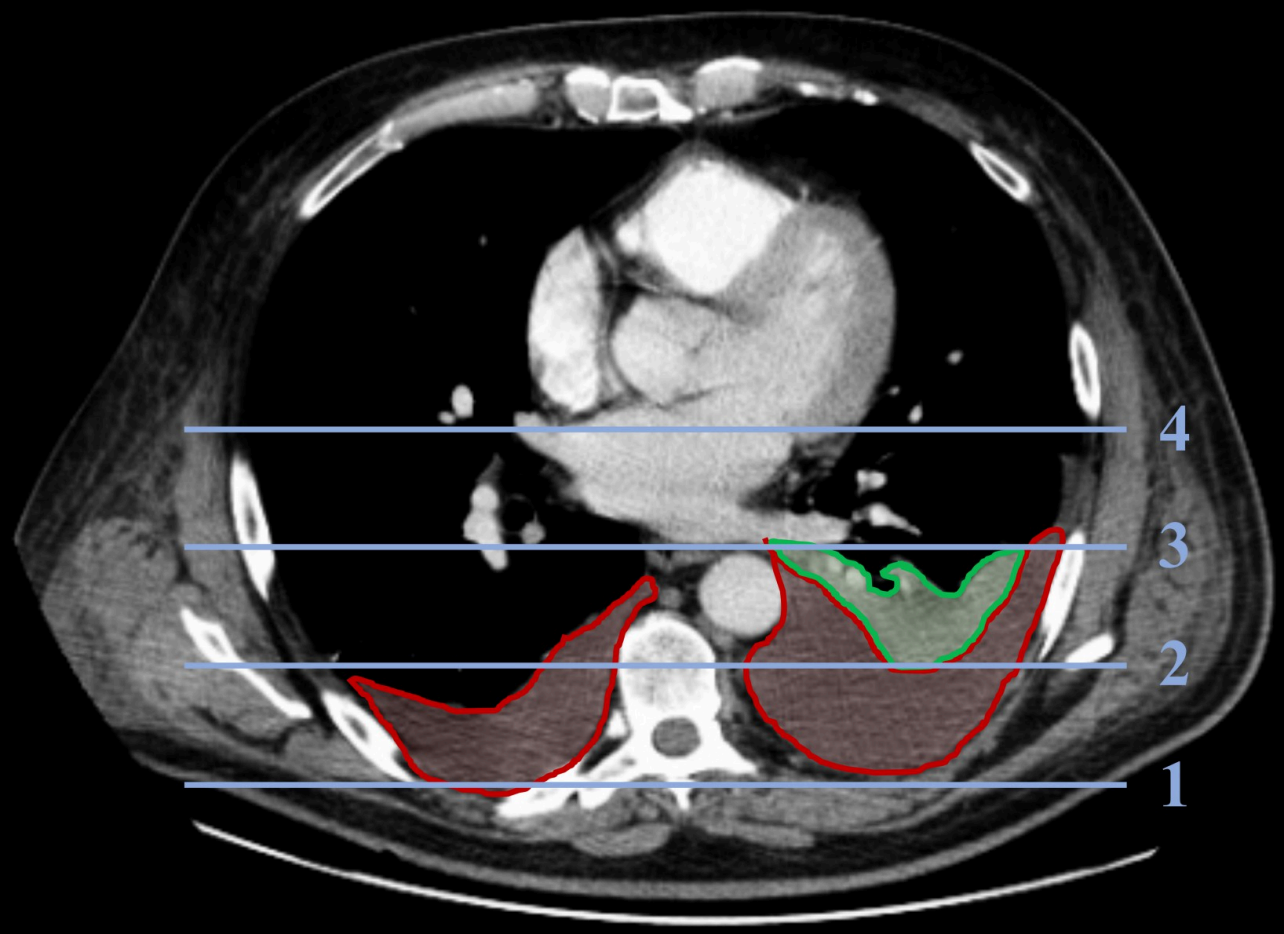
Exemplary representation of CECT imaging (mediastinal window) with unilateral dystelectasis (green) and severe, bilateral pleural effusions (red). Pleuropulmonary changes were analysed in the caudal sections of the thorax captured on the abdominal CECT (contrast enhanced computed tomography) scan. Thorax CECTs were additionally screened for pathologies, if available. The size of pleural effusions was determined in the transverse plane of the lung and mediastinal window. The area dorsal the midline of the thorax was divided into three parts similar in size (blue lines). Pleural effusions were classified as “low” if they remained dorsal line 2, as “moderate” if exceeding line 2, but not line 3 and as “severe” if exceeding line 3.
